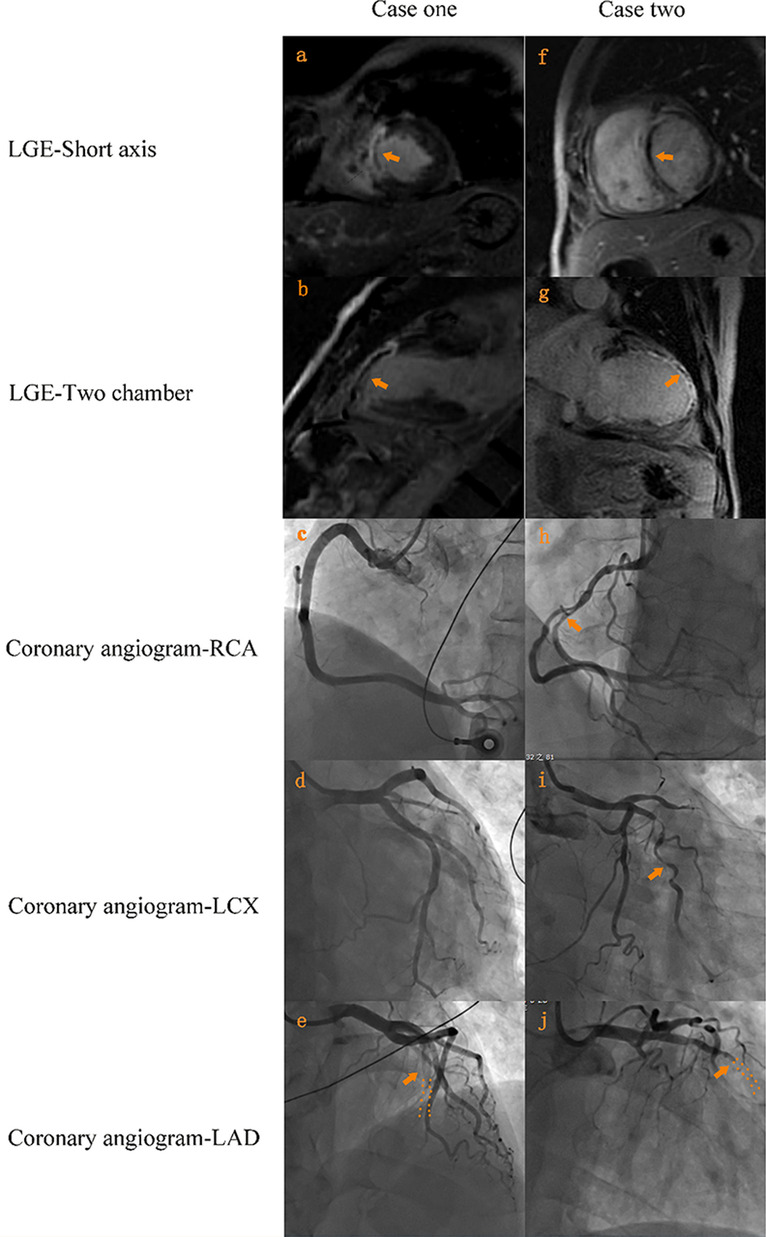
Two case examples of arteriography and cardiac magnetic resonance image. Case one (a–e): A 59 years old man with anterior ST-elevation myocardial infarction was identified to have a single total occluded LAD (SYNTAX score 18.5 and infarct size 22.74); Case two (f–j): A 64 years old man also with anterior ST-elevation myocardial infarction was identified to have total occluded LAD and diffused diseased intermediate branch and RCA (SYNTAX score 32.5 and infarct size 54.91). *LAD* left anterior descending artery; *RCA* right coronary artery
